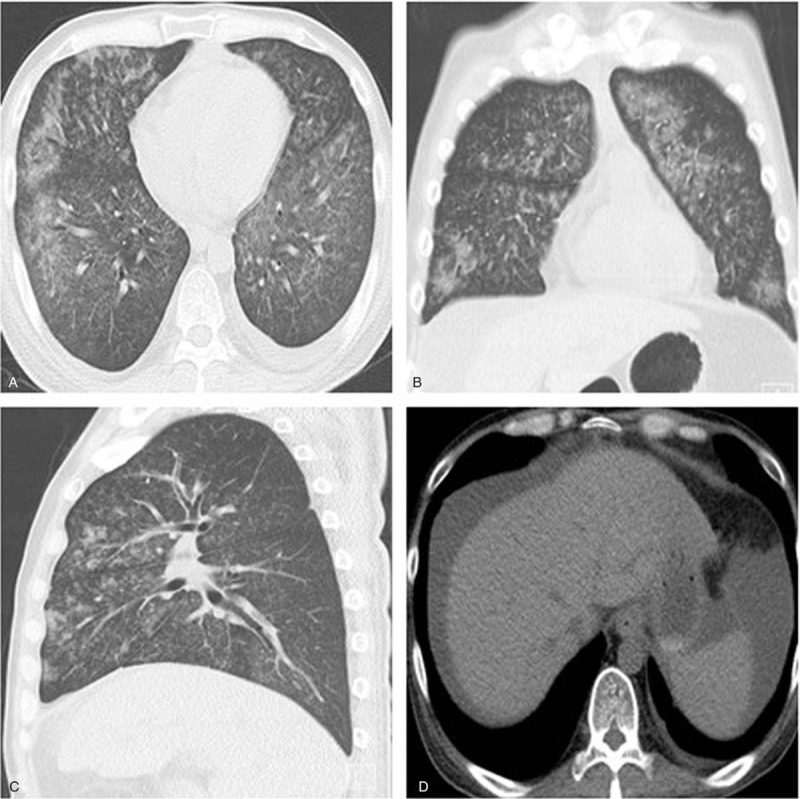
Plain axial CT scan and multiplanar reconstruction showing multiple patchy ground-glass opacities in both lungs with unclear boundaries. Changes in the air-like cavities were observed in both lungs, showing lobular or subsegmental distribution where air bronchograms were observed.
